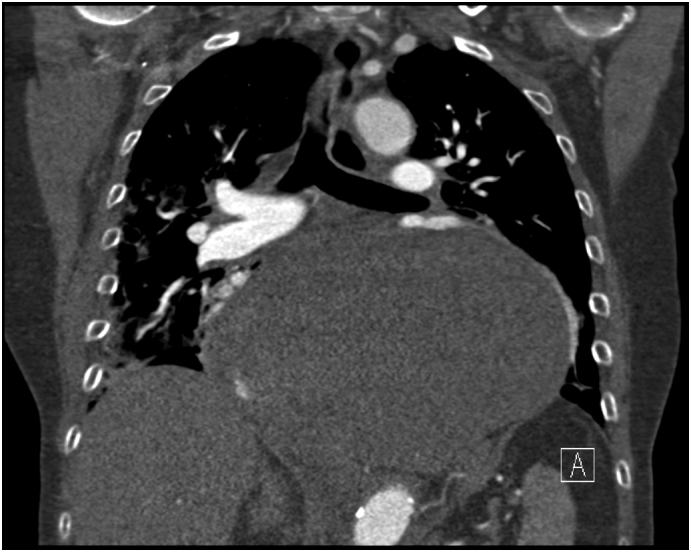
CT of mixed hiatal hernia - coronal section.
